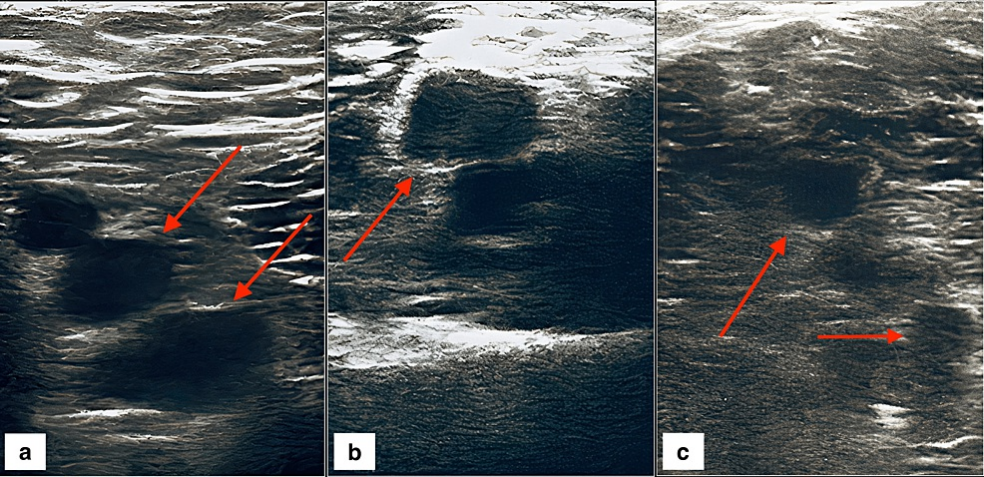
Thrombotic findings on ultrasound imaging upon the patient's admission. All images were taken during transducer compression of the veins. The inability to compress the vein indicates the presence of venous thrombi. Figure 1A shows non-compressible left femoral (upper arrow) and deep femoral (lower arrow) veins. Figure 1B shows the non-compressible right popliteal vein (arrow). Figure 1C shows non-compressible posterior tibial veins (arrow). Unlabeled lumens in all three images represent arteries.
